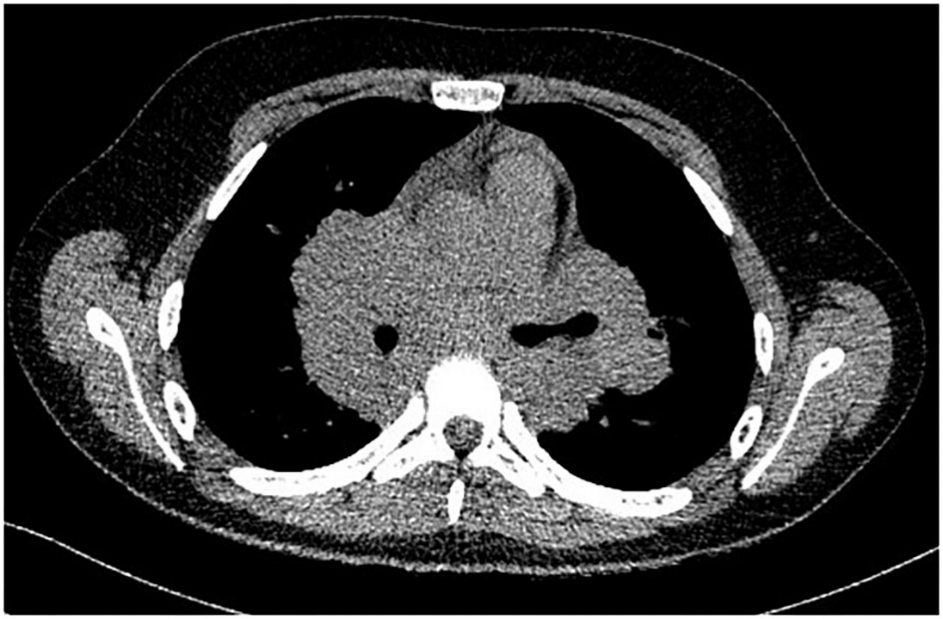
CT aspect of mediastinal lymphadenopathies.
